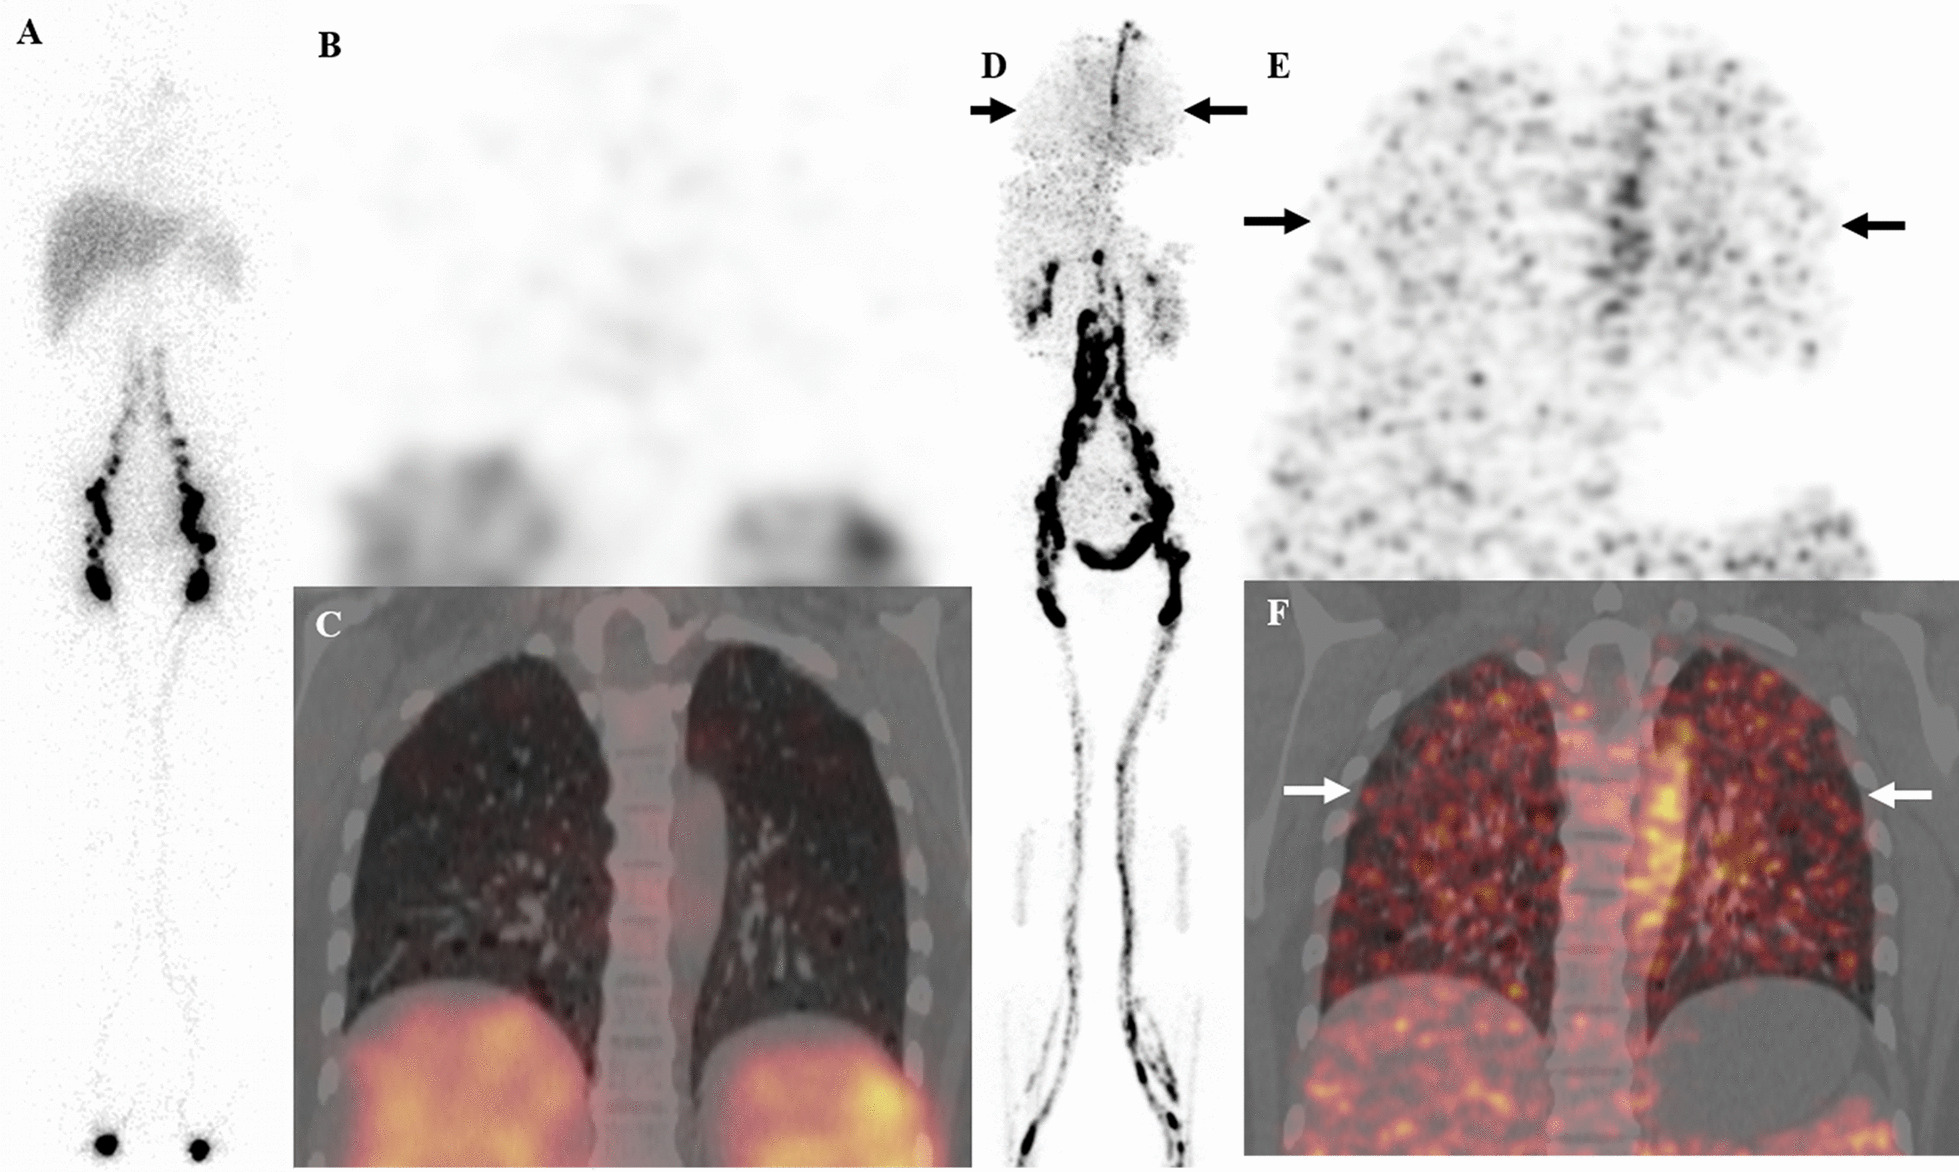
A 34-year-old woman (patient #7) with LAM. The ^99m^Tc-ASC lymphoscintigraphy (A) and SPECT/CT (B, SPECT; C, fusion) at 1 h after tracer injection appeared negative. However, on ^68^Ga-NEB MIP image (D, arrows), there was abnormal, increased activity in the chest. And coronal images (E, PET; F, fusion; arrows) of the chest revealed that the chest activity was in the lung. No extrapulmonary lymphatic manifestations were found on ^68^Ga-NEB PET/CT
